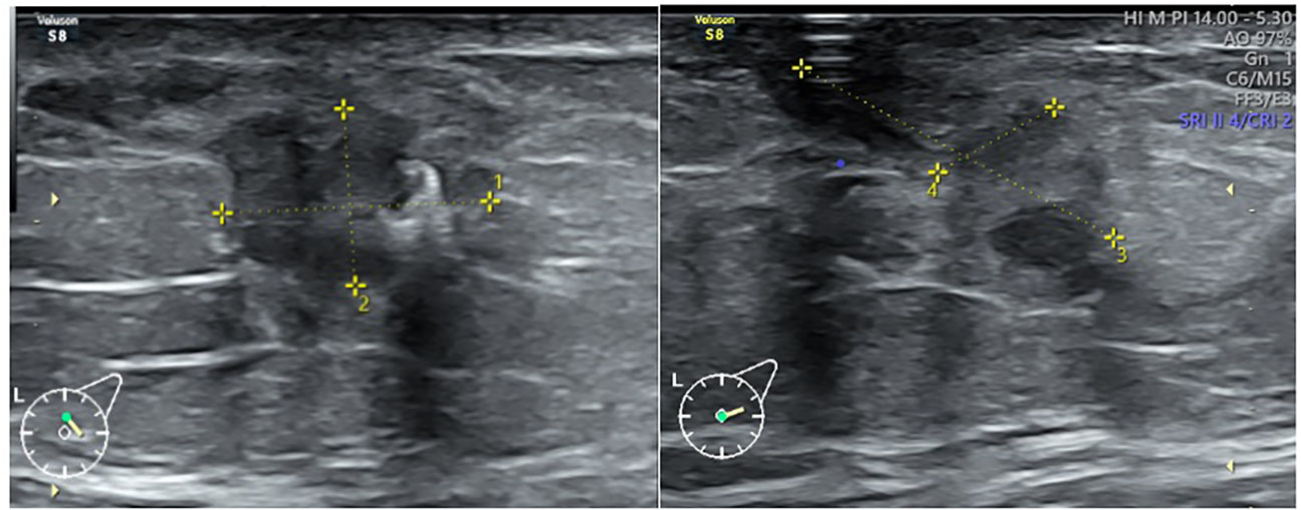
Ultrasound images of the left breast from two different angles. The predominantly hypoechoic mass lesion with irregular shape is visible. Maximum dimensions measured were 13.15 mm (1), 8.50 mm (2), 18 mm (3), and 6.77 mm (4).
